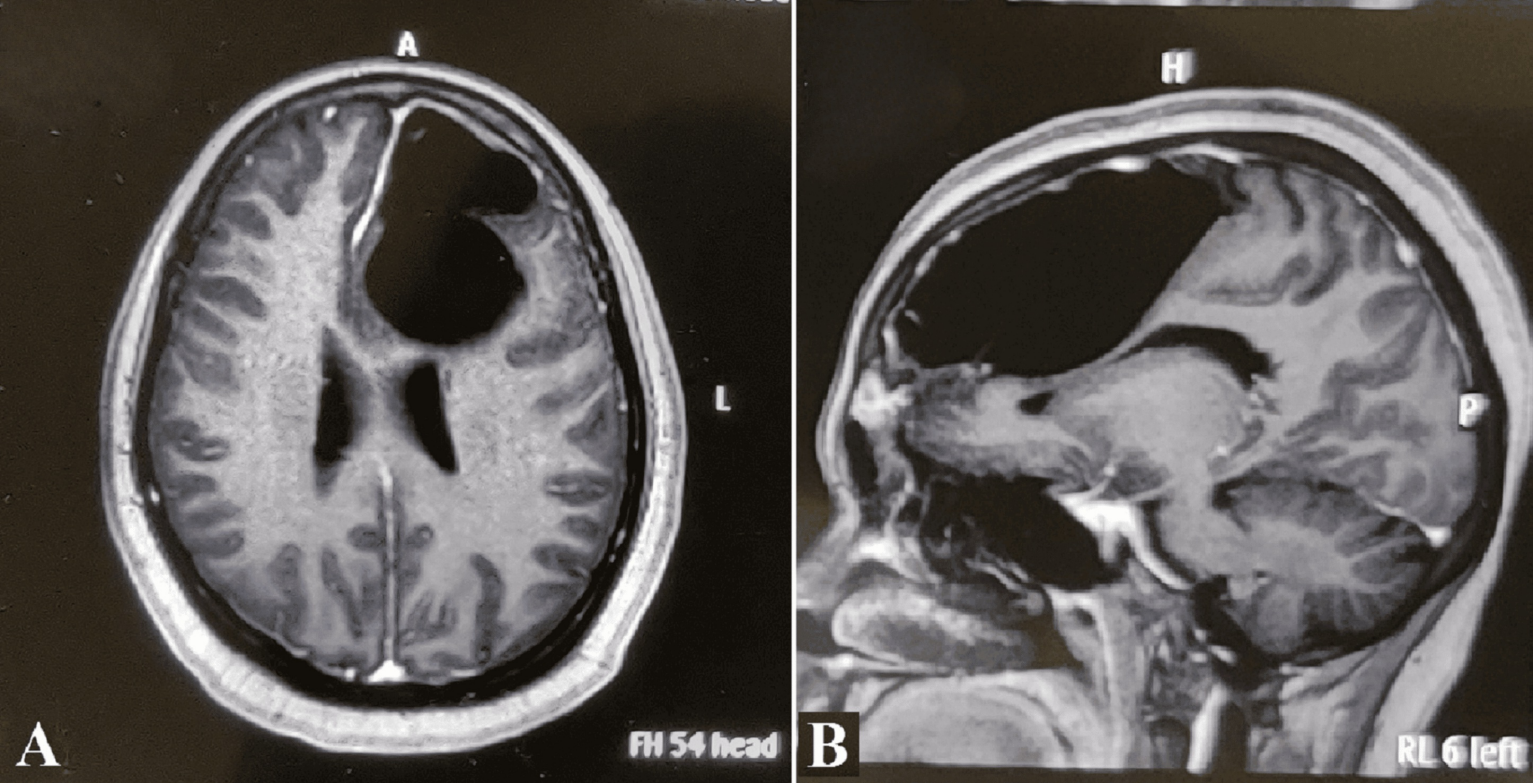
MRI brain T2-weighted axial image (A) and T1-weighted postcontrast sagittal image (B) reveal an isointense osseous lesion in the left frontal sinus, associated pneumocephalus without peripheral enhancement, and a mass effect on the left frontal lobe MRI: magnetic resonance imaging
